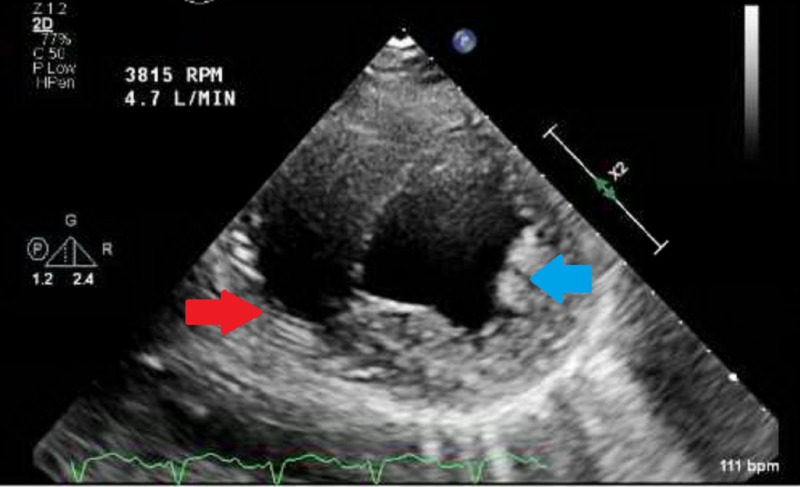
Transthoracic echocardiogram with severely dilated right ventricle (red arrow) and left ventricle (blue arrow) in short axis view
